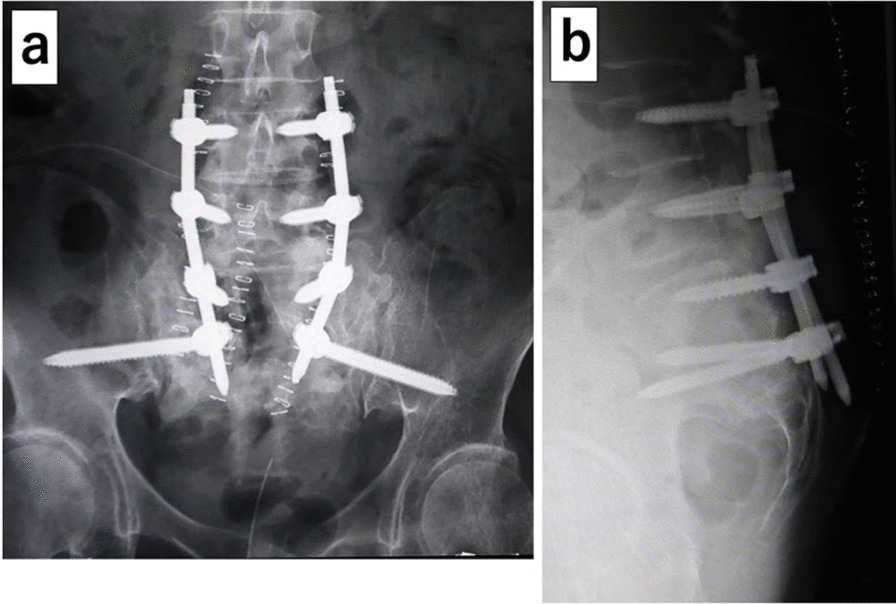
Postoperative radiographs. a Anteroposterior image; b lateral image. First to third sacral laminectomy and alar–iliac fixation using percutaneous pedicle screws and sacral alar–iliac screws have been performed
